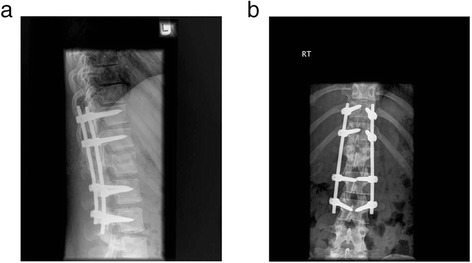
Radiograph showing stabilisation and degree of kyphosis correction a. Lateral b. Anterio-posterior.
